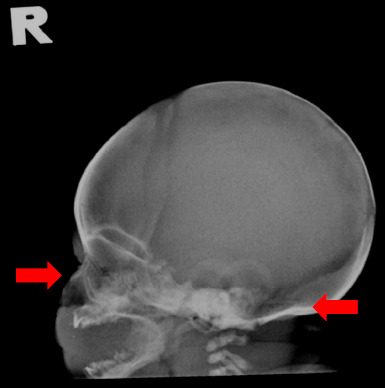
Lateral skull radiograph showing a small skull base and a depressed nasal bridge.
